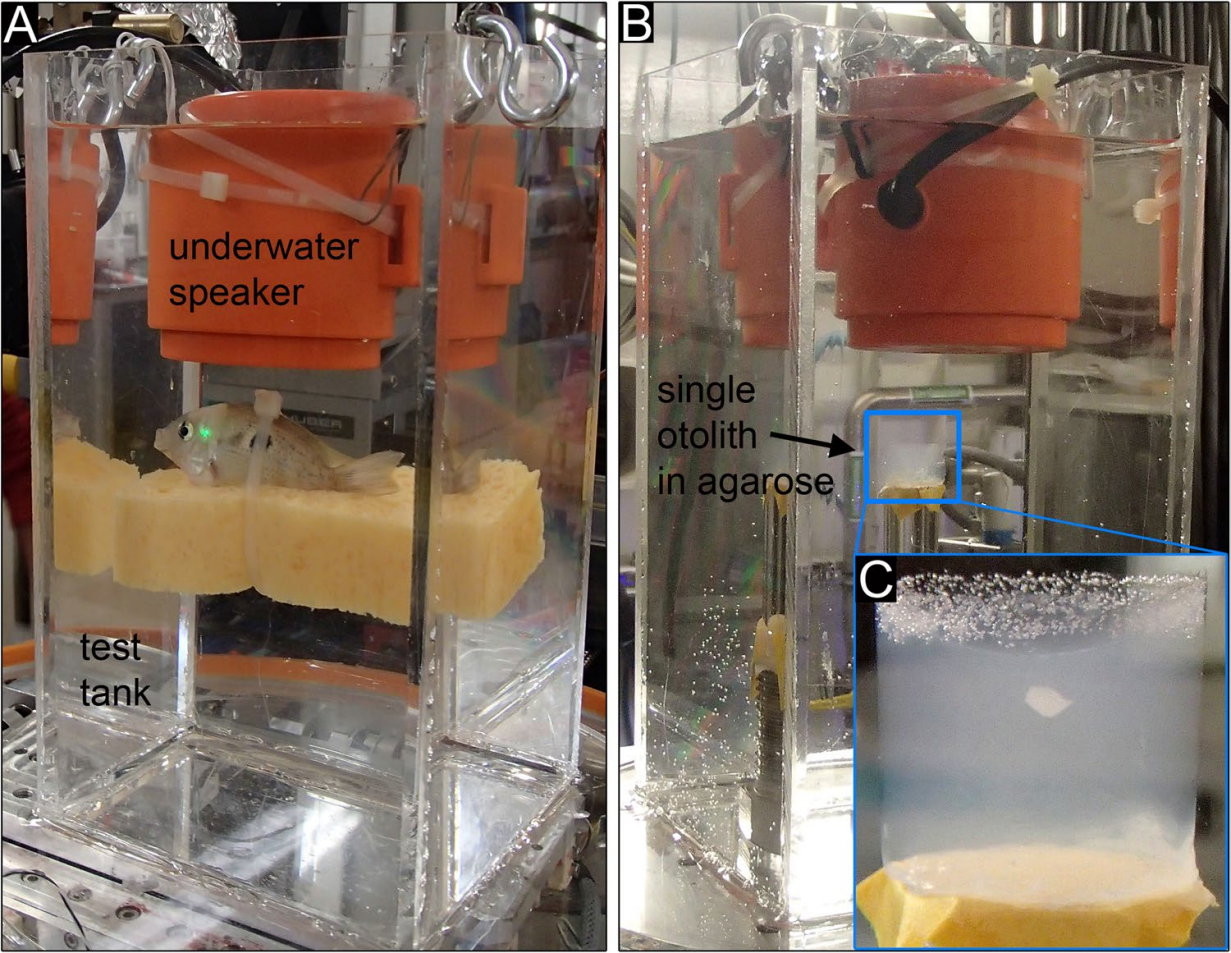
Set-up for the 2D radiographic experiments using (A) a fresh-dead fish or (B) an isolated otolith embedded in 1% agarose as test subjects. The inset in (C) shows the isolated right saccular otolith of *E*. *maculatus* with sound impinging on the dorsal otolith margin.
